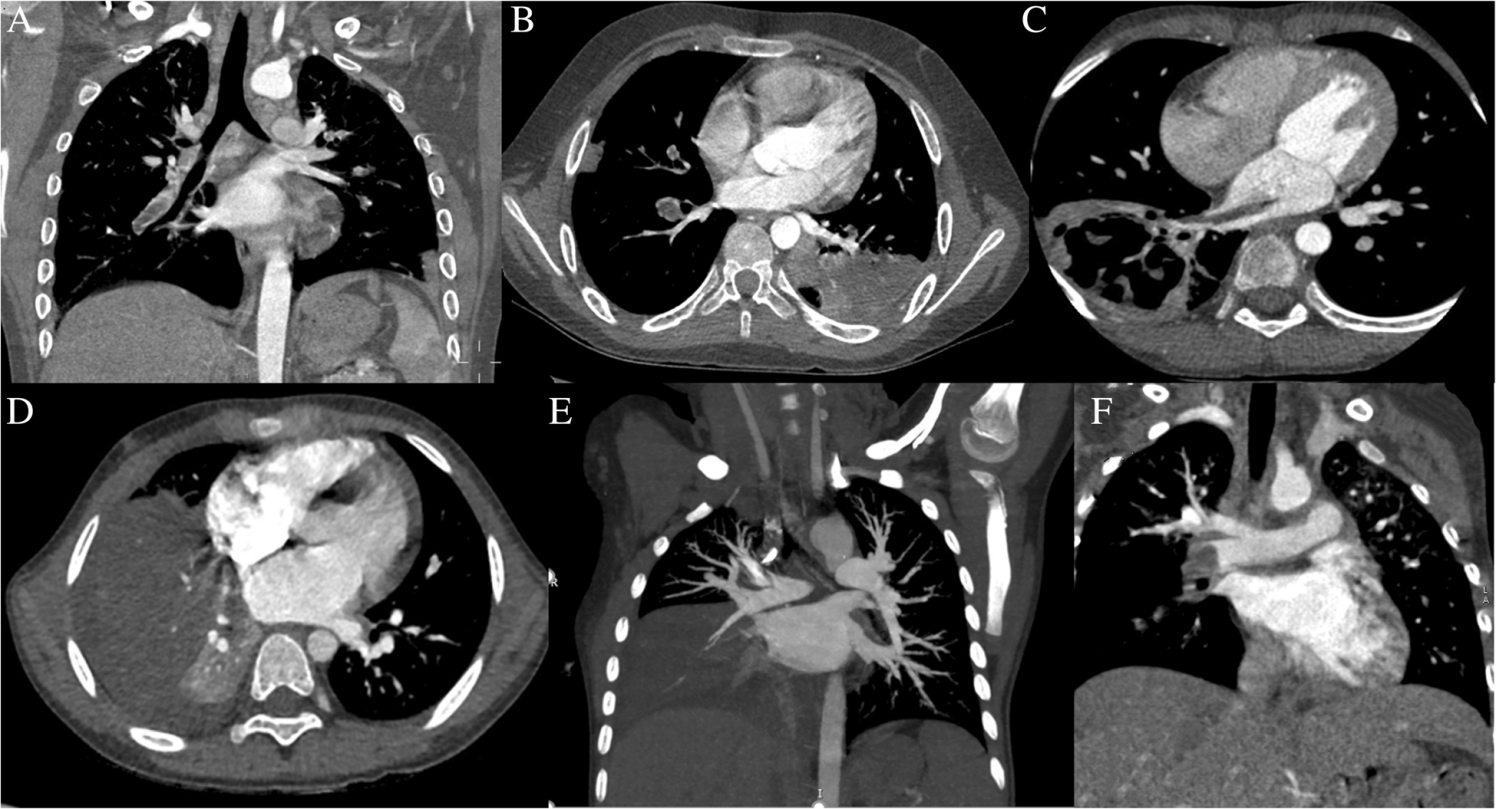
Lung enhanced CT and 3D vascular reconstruction revealed right lower pulmonary artery thrombosis (a, b), necrosis within consolidation of the left lower lung (b), low-density necrosis of the right lower lung with cavity formation, and the strip filling defect in the right lower pulmonary vein extended to the left atrium (c), necrosis of the right lower lung (d) with right lower pulmonary vein occlusion (d, e), and right pulmonary artery thrombosis (f)
